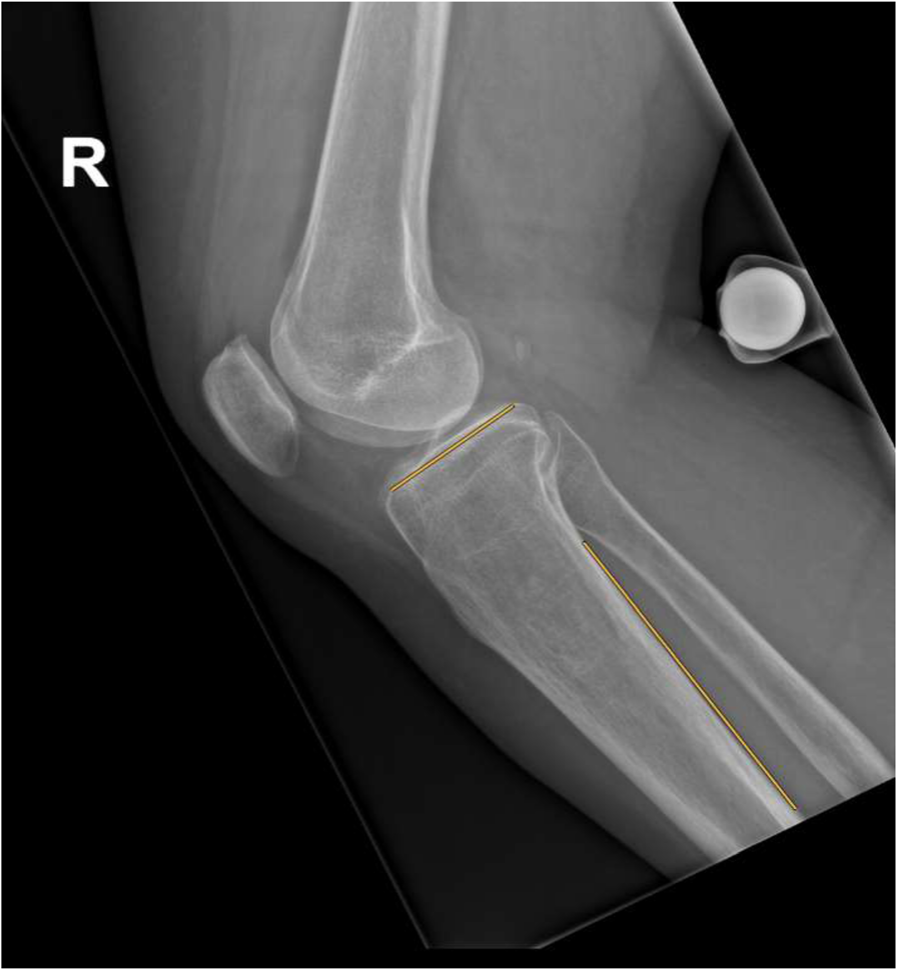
Lateral view radiograph of a right knee with the two lines (orange) for conventional measurement of the posterior tibial slope
